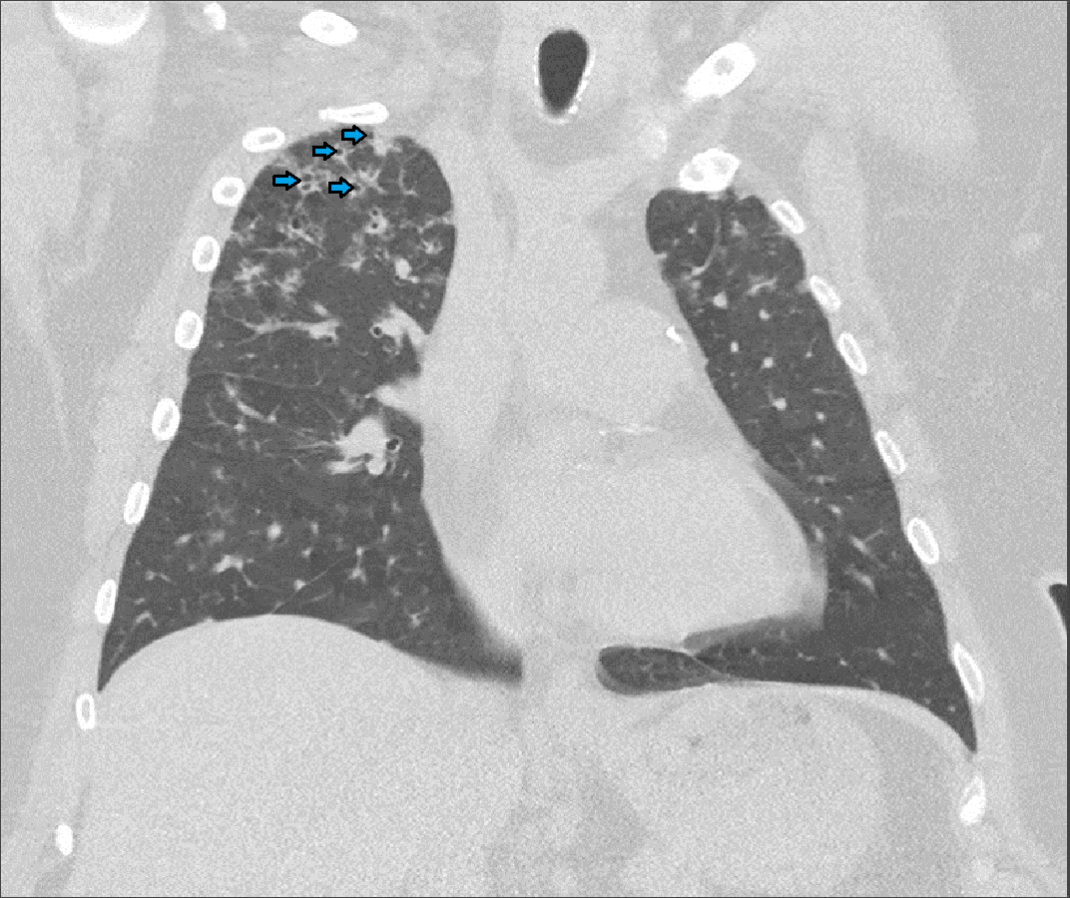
Computed tomography of the chest after three months of antimycobacterial therapy showing development of more cavitary lesions
